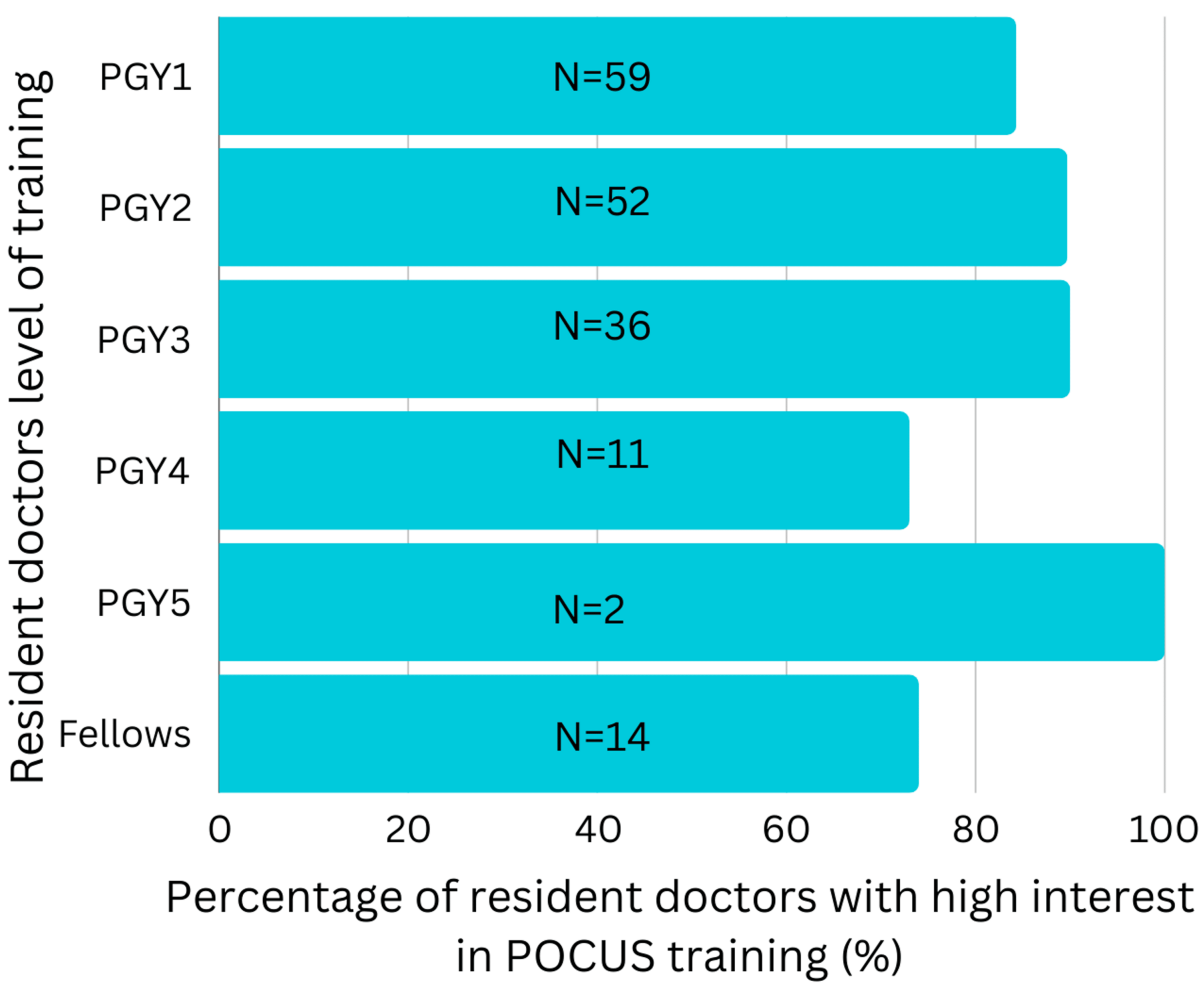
Percentage of resident doctors with high interest in POCUS training. POCUS: Point-of-Care Ultrasound; PGY: Post-graduate year.
